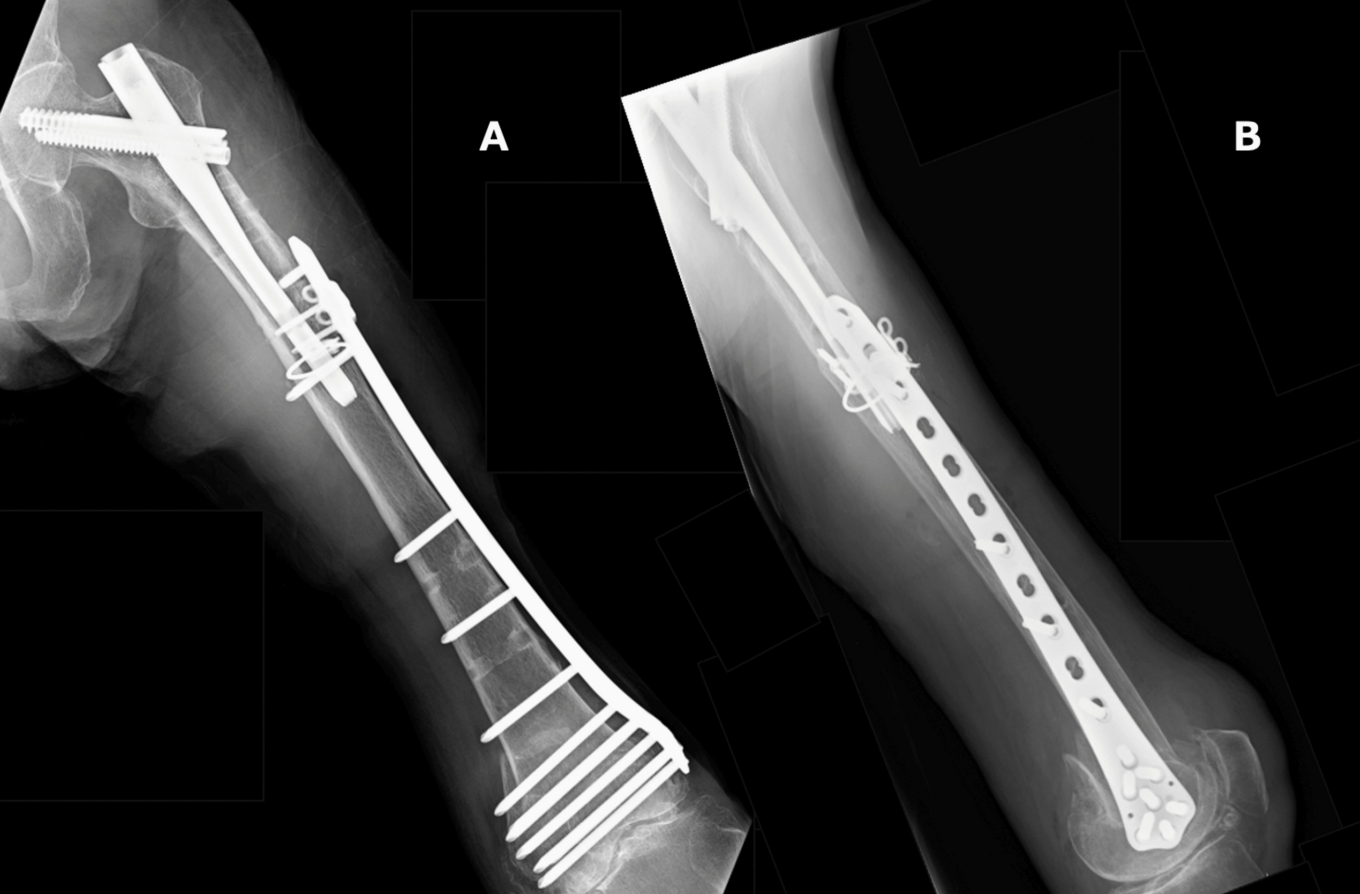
Radiograph findings A: postoperative anteroposterior radiograph; B: postoperative lateral radiograph
